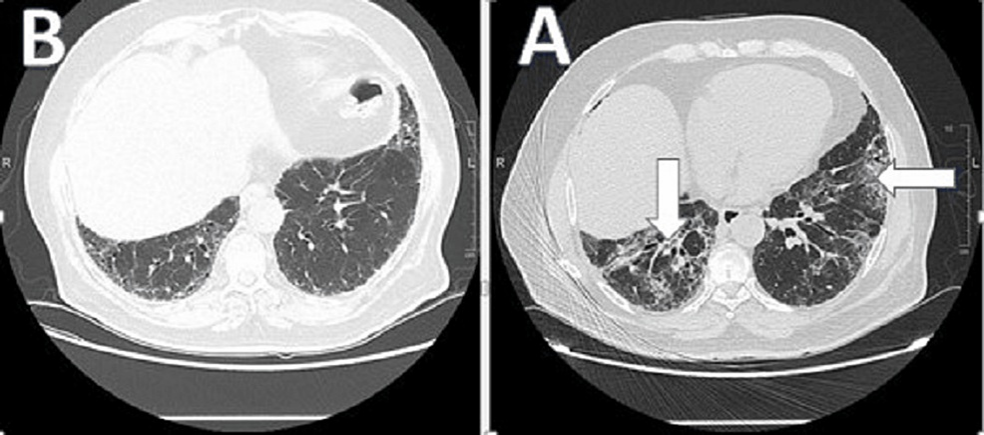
CT of the chest shows the changes before the intervention (A) and the same cut of chest CT scan six months after intervention (B)
